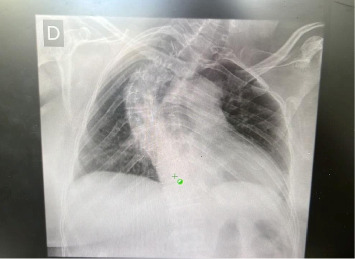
Chest X-ray demonstrated severe scoliosis and alterations in the rib cage.
